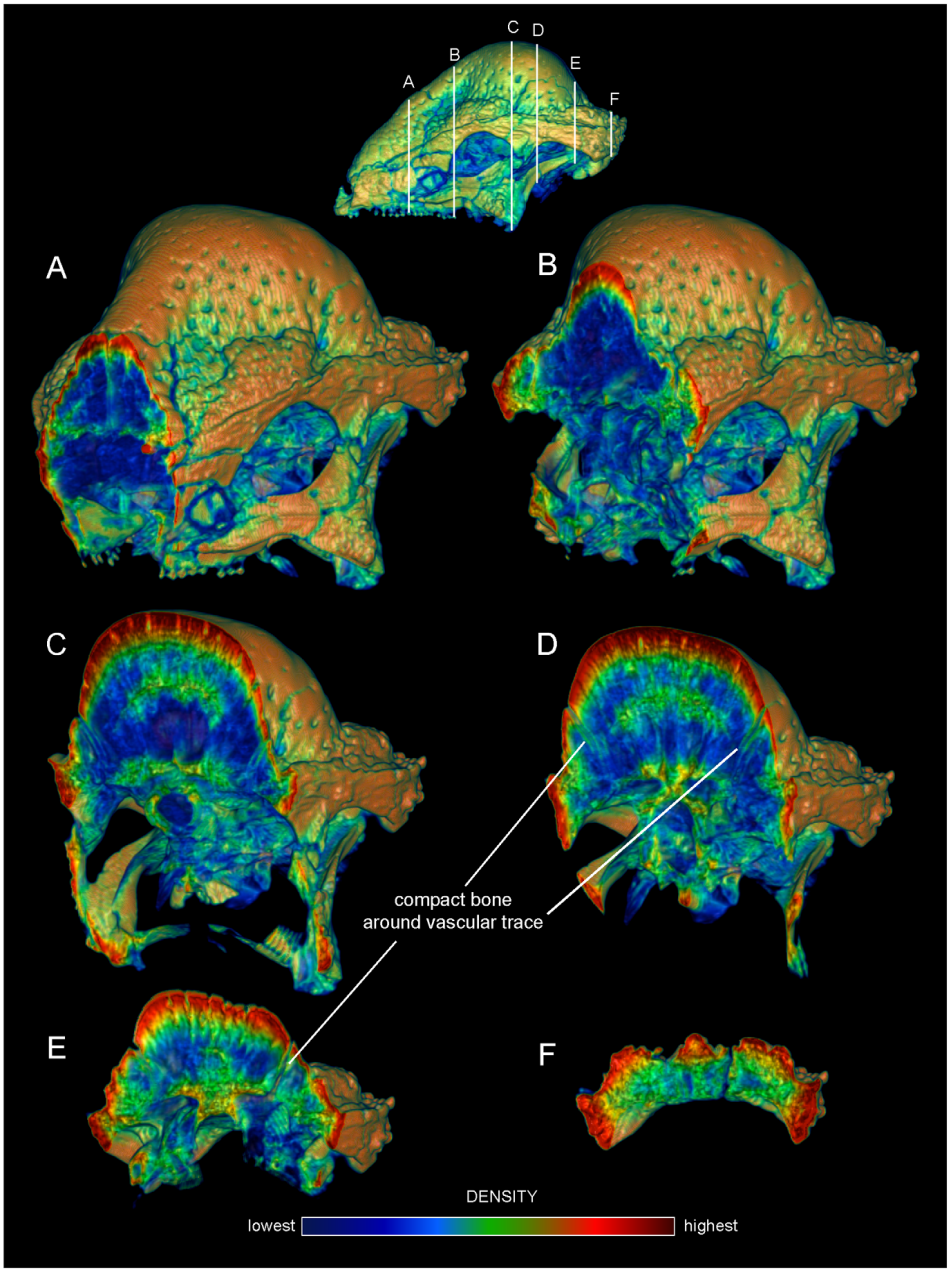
Internal densities of bone in *Stegoceras validum* (UA 2). Transverse CT sections from anterior (A) to posterior (F) through the cranium of *Stegoceras validum* (UA 2) seen in anterior oblique view. Inset CT reconstruction in lateral view (top) depicts section positions. Density and thickness of cortical bone increase towards the apex of the dome from the periphery, anteroposteriorly (B–D) and medially (C, D). Trabeculae radiate roughly perpencidular to the dome's outer surface, evident in the low-density (blue) region posterior to the orbit (B–E). Note that density of superficial bone may be inflated by beam hardening, but a dense, deep compact layer is definitively present. Dense compact bone (Hounsfield values of approximately 2000) surrounds presumed vascular traces, forming tubes that empty onto the dome surface; three of these are visible in D and E. These tubular structures recall struts within artiodactyl cranial sinuses.
